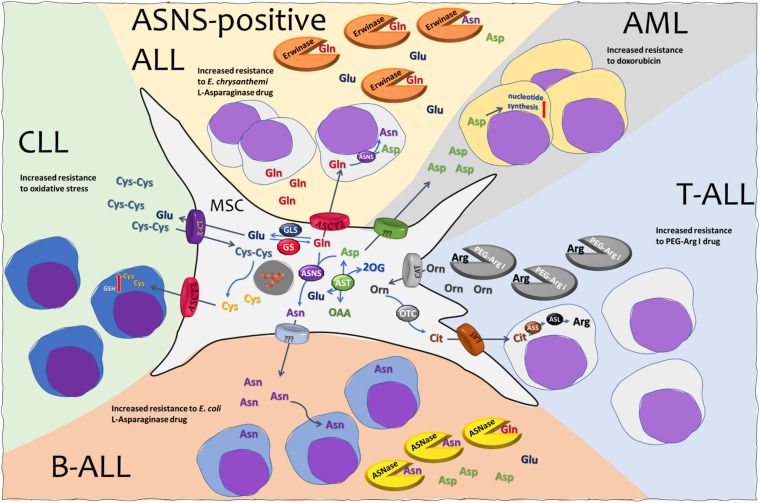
Mesenchymal stromal cells (MSCs) and amino acid crosstalk in leukemia. See text for explanation. MSC, mesenchymal stromal cell; ALL, acute lymphoblastic leukemia; AML, acute myeloid leukemia; CLL, chronic lymphocytic leukemia; ASNS, asparagine synthetase; ASL, argininosuccinate lyase; ASS, argininosuccinate synthetase; AST, aspartate aminotransferase; OTC, ornithine transcarbamylase; GLS, glutaminase; GS, glutamine synthetase; ASNase, l-asparaginase from *E. coli*. Erwinase, l-asparaginase from *E. chrysanthemi*; PEG-Arg I, PEGylated recombinant human arginase I; ASCT2, alanine serine cysteine transporter 2; CAT, cationic amino acid transporter; LAT, large neutral amino acid transporter; X-CT, cystine/glutamate transporter.
